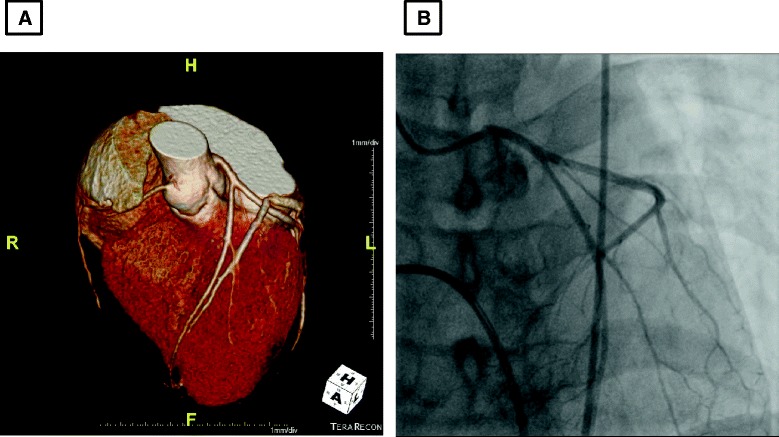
Coronary artery image demonstrating a hypoplastic right coronary artery without significant luminal narrowing of major vessels. A: Coronary computed tomography angiography; B: coronary angiography showed no coronary artery lesion.
